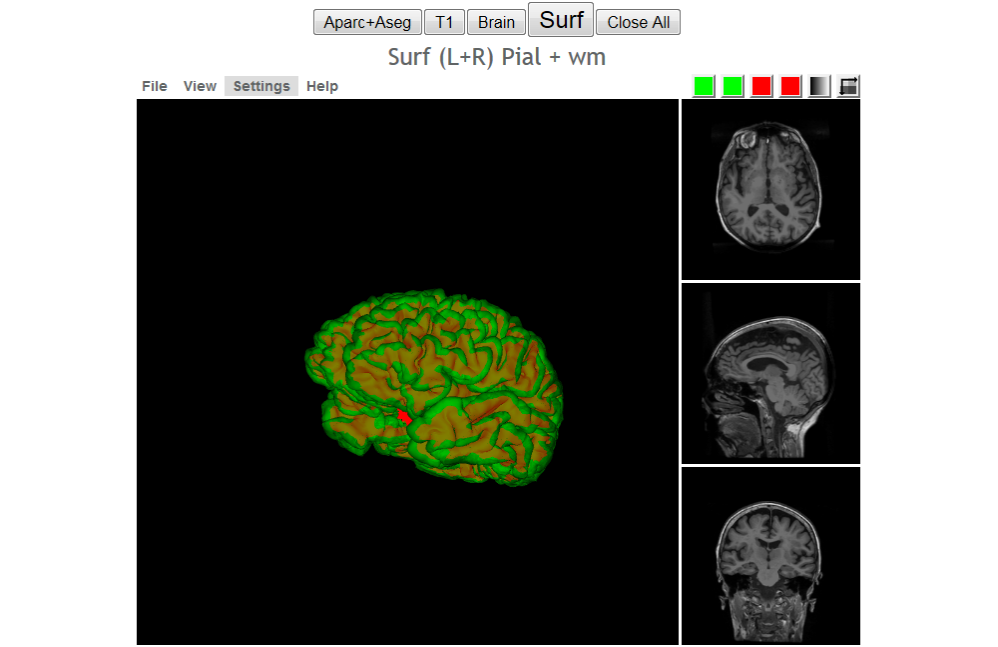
Interactive viewer, showing the surface reconstruction of and anatomical magnetic resonance imaging (MRI), Green represents the gray matter, Red represents the white matter.
